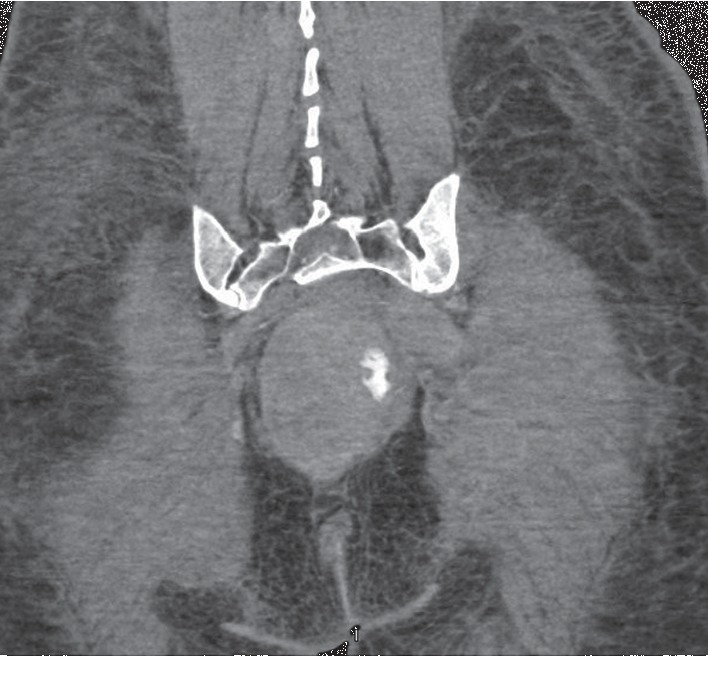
Sagittal view of CT angiogram showing active extravasation of contrast in the territory of the left internal iliac artery and inferior rectal artery.
